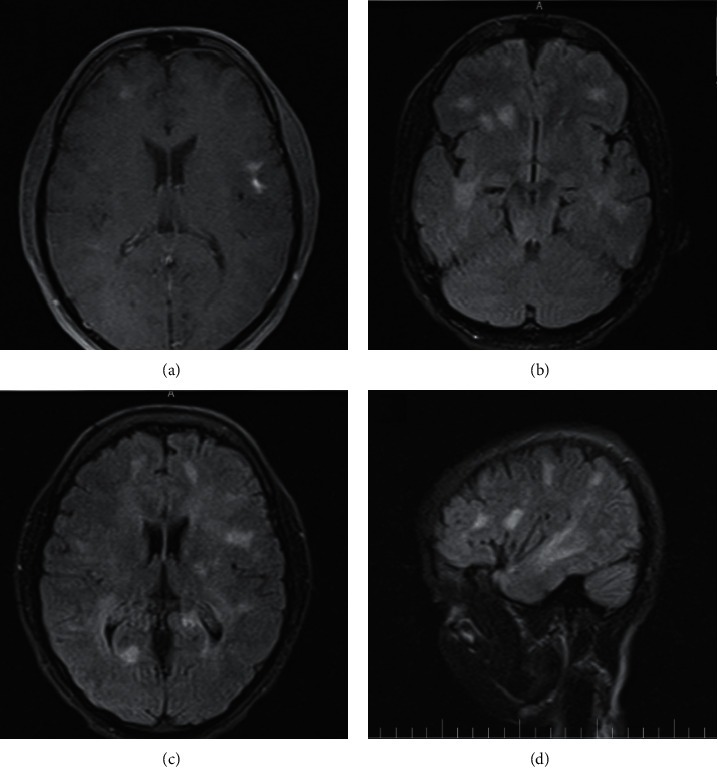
(a) Head-MRI T1 sequence with gadolinium demonstrates various hypersignal lesions in favor of active demyelinating plaques in left temporal and right frontal areas of the brain. (b, c) Axial and (d) sagittal FLAIR views show plaques with larger sizes in deeper areas of the brain.
